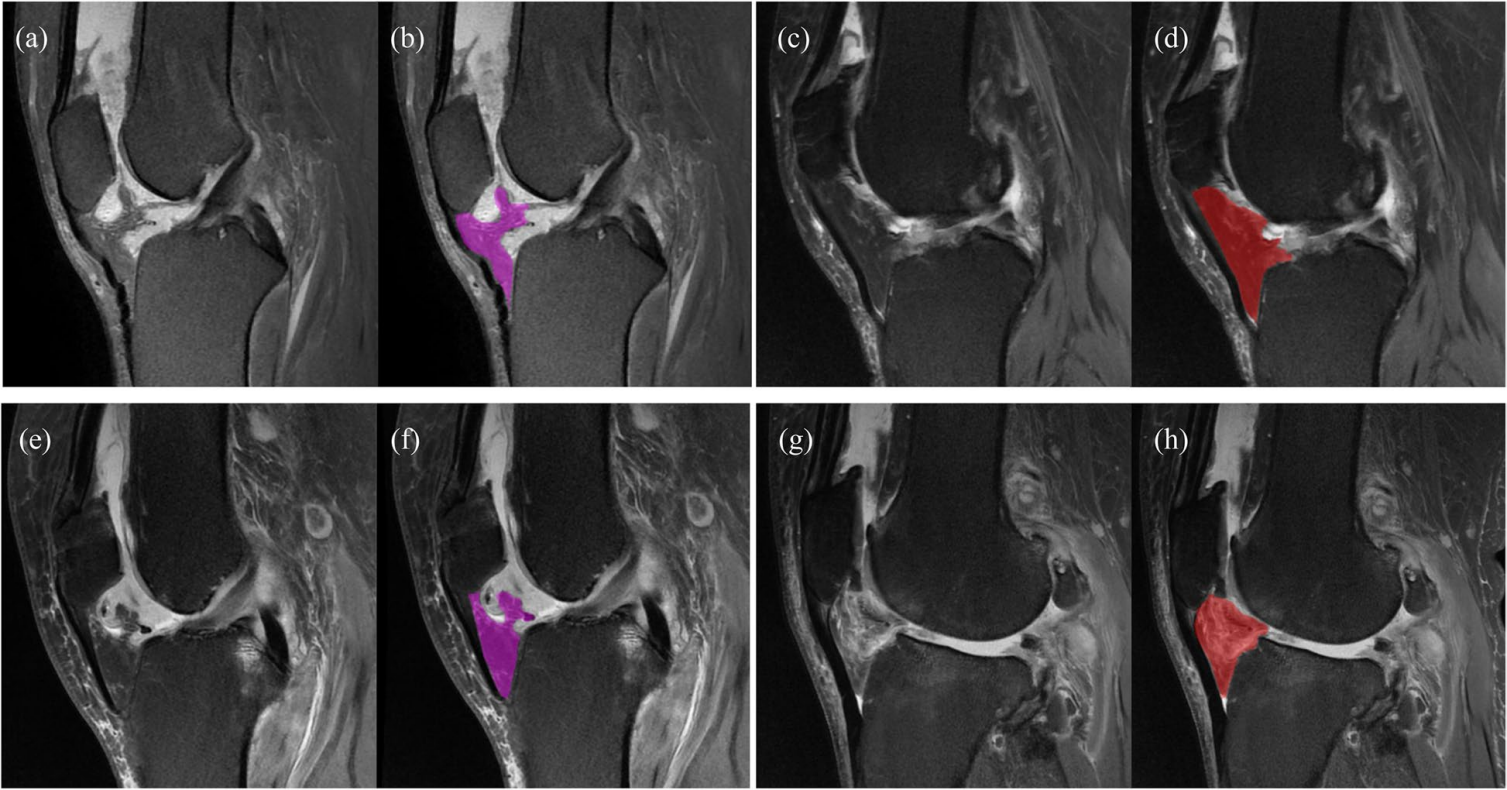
MRI images of two pairs of patients in our study cohort. The two aligned channels of four patient data (for visualization purpose, we only show the middle sagittal (mid-sagittal) slice of each channel. Purple and red masks are manual ROI for infrapatellar fat pad in patients with rheumatoid and knee osteoarthritis, respectively. a–d Two 54-year-old male patients with knee pain. a, b: RA showed positive broken-fat pad (BFP) sign. c, d OA showed negative BFP. e–h Two 66-year-old female patients with limited knee function. RA patients (e–f) showed positive BFP signs, and OA patients (g–h) showed negative signs
